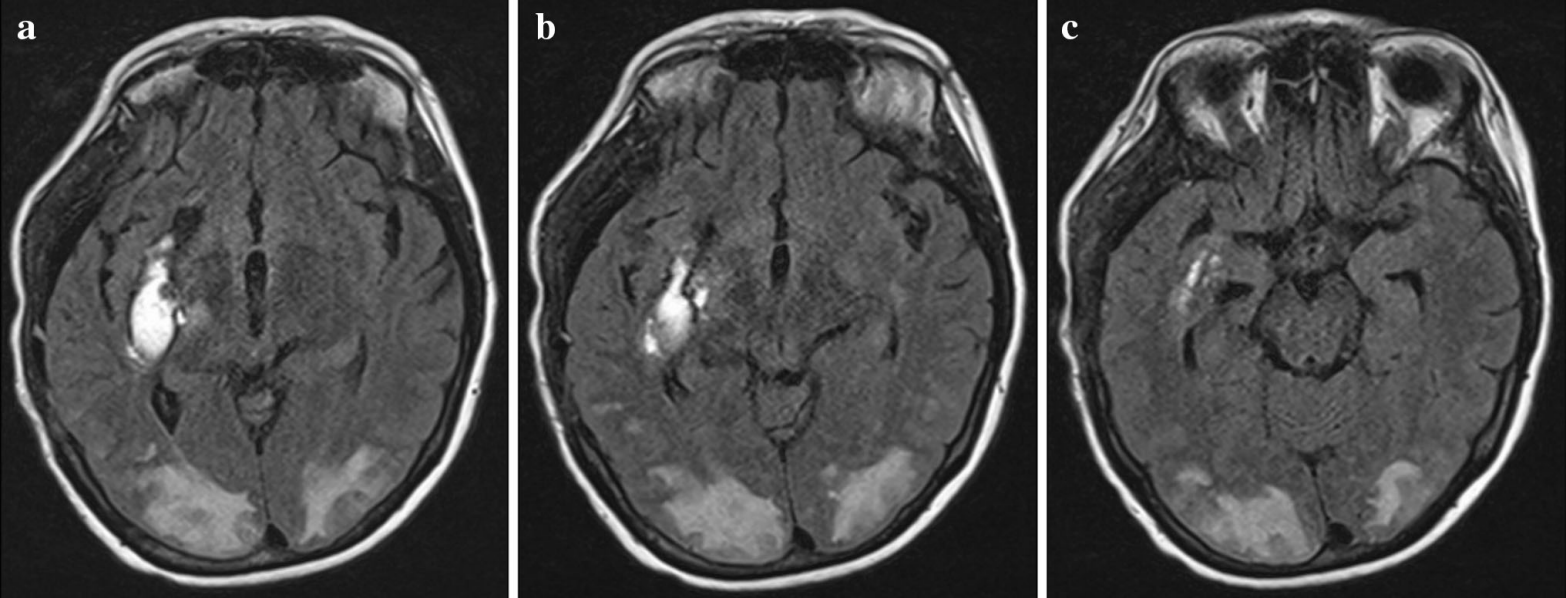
a–c Axial MR image (fluid-attenuated inversion recovery sequence) demonstrates extensive vasogenic edema in the occipital region bilaterally and right insular hemorrhage
